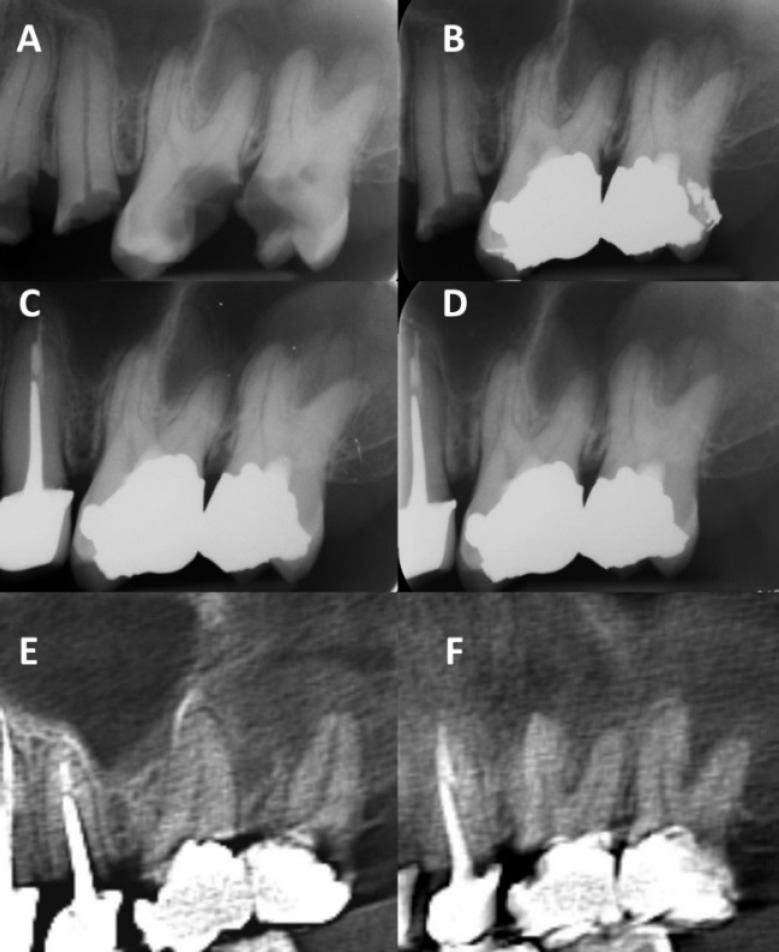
A)* Preoperative periapical radiograph. Note the extensive caries in molars and normal periodontium on all roots; *B)* Immediate postoperative radiograph; *C)* One-year recall; *D)* Two-year recall. Note the normal PDL space and normal radiographic view of the roots and canals (no internal/external root resorptions); *E)* CBCT view, sagittal section on palatal roots #14 and #15; *F)* CBCT view, sagittal section on buccal roots #14 and #15 showing normal periodontium
